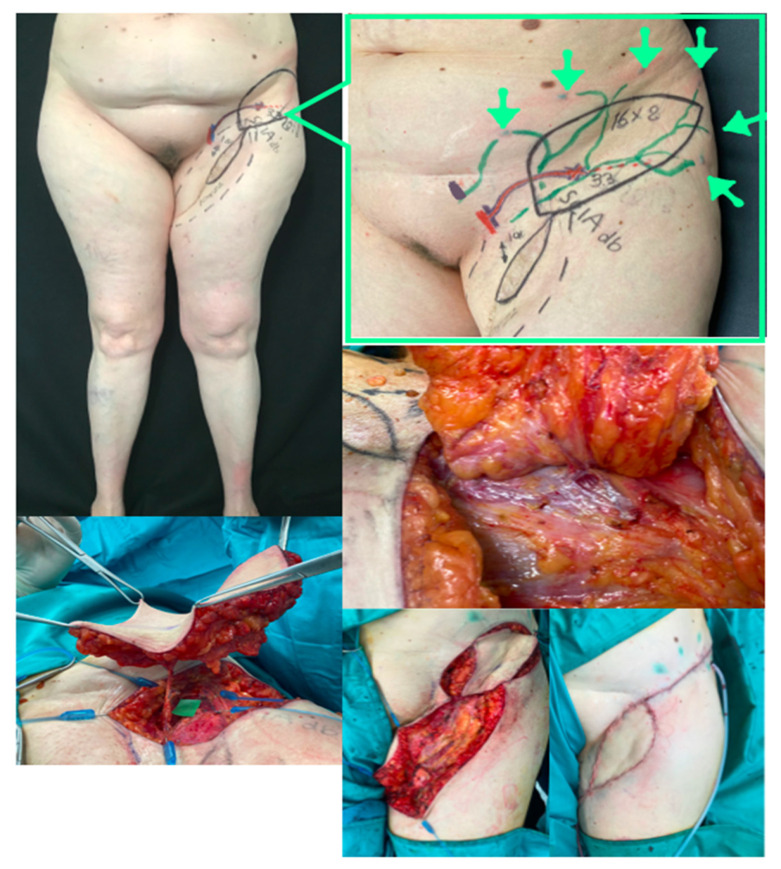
LIFT case example. Above, left: Preoperative picture of 57-year old female patient suffering from a retracted inguinal and upper thigh scar three years after inguinal dissection for melanoma of the foot. Lymphoscintigraphy showed dermal backflow in the upper inner thigh. Above, right: Preoperative planning markings performed with US and ICG-lymphography. Notice that in addition to the long inguinal scar, we found that the SCIA deep branch was spared, travelling below the muscular fascia on top of sartorius muscle and perforating the fascia at the marked point. Perforator calibre was 3.3 mm (probably due to delay phenomenon connected to ligation of other superficial vessels during inguinal dissection). Green arrows show the ICG injection points to highlight the lymphatic channels draining in some residual upper deep pelvic–inguinal lymph nodes. The skin paddle is marked in black. Middle, right: Flap elevation shows that the big perforator matches exactly with preoperative planning in terms of position, fascia perforation point and calibre. Below, left: The flap is elevated and the SCIA dissected up to its origin. Below, right: The thigh scar was revised and the flap rotated and transposed for final inset.
